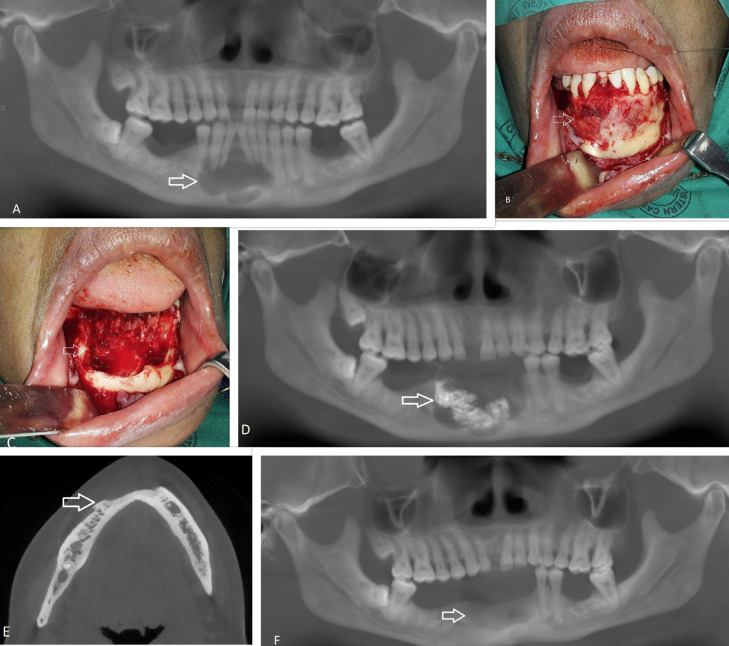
A: Panorex showing multilocular Glandular Odontogenic Cyst (GOC) in anterior mandible. B: Intra-operative image of lesion. C: Defect post enucleation of lesion. D: Day one post-operative panorex with 5-Fluoruracil soak gauze in the surgical defect. E: Axial CBCT 14 months post treatment showing new bone deposition and no sign of recurrence. F: Panorex 14 months post treatment with good bone filling in defect.
